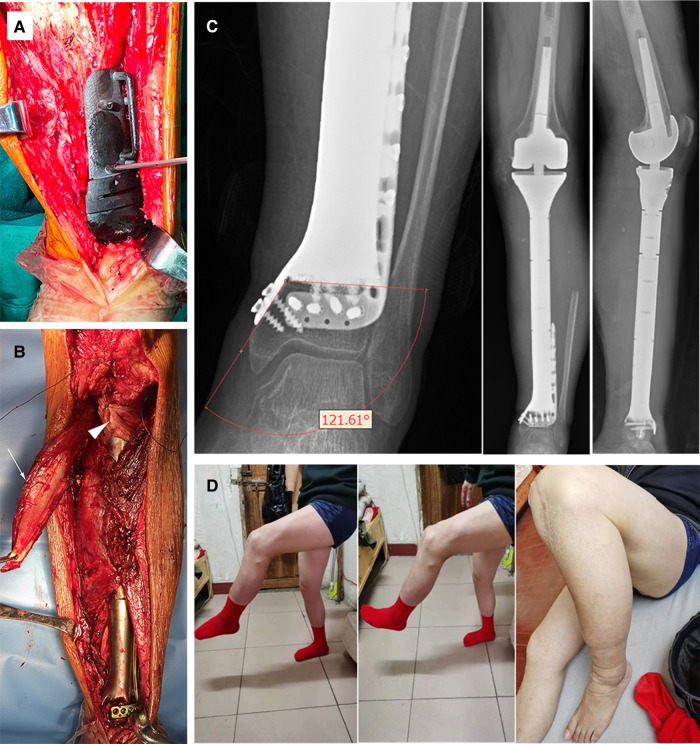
(A) Intraoperative utilization of PSG to ensure precise osteotomy; (B) The anatomic implantation of the prosthesis. White arrowhead: medial gastrocnemius flap; triangular arrowhead: double-surface reconstruction of the knee extension mechanism with an allogenic tendon. (C) X-ray of the lower limb 12 months after surgery. The angle of the medial plate was about 121 degrees, which was consistent with the design. No sign of ischemic necrosis or collapse of the distal ultra-small bone fragment was observed. (D) The active knee range of motion (ROM) was 0–105 degrees and the patient sustained active ankle ROM of dorsal flexion 0–15 degrees, and plantar flexion 0–45 degrees at the latest follow-up.
